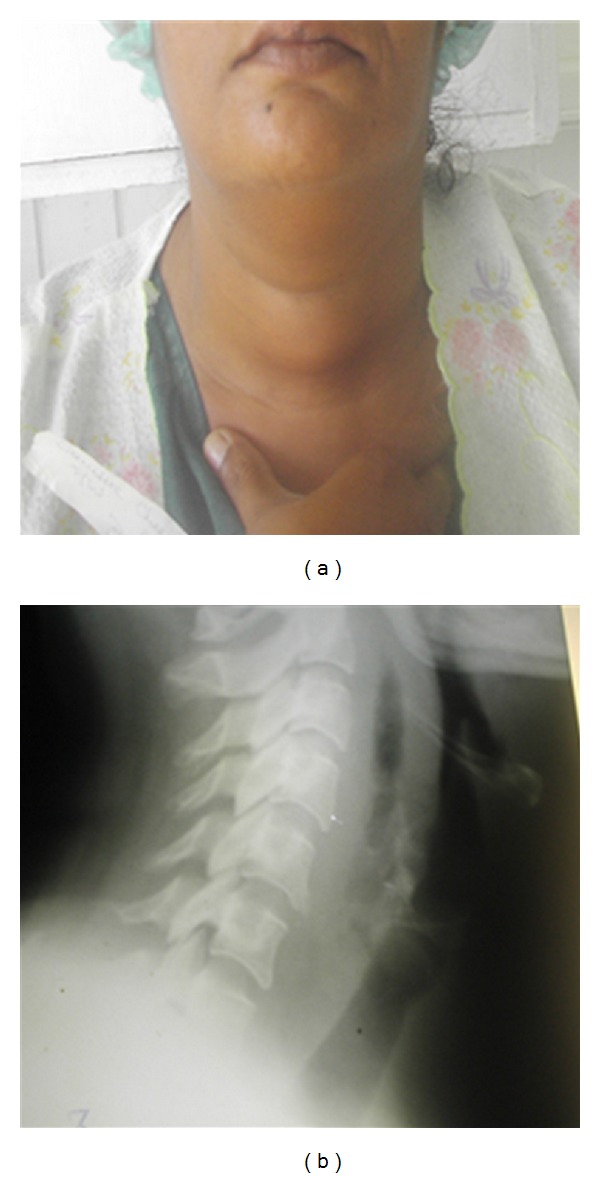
A photograph demonstrating the central neck swelling (a). Lateral radiograph of the same patient showing extensive retropharyngeal thickening with anterior hyperdensity.
